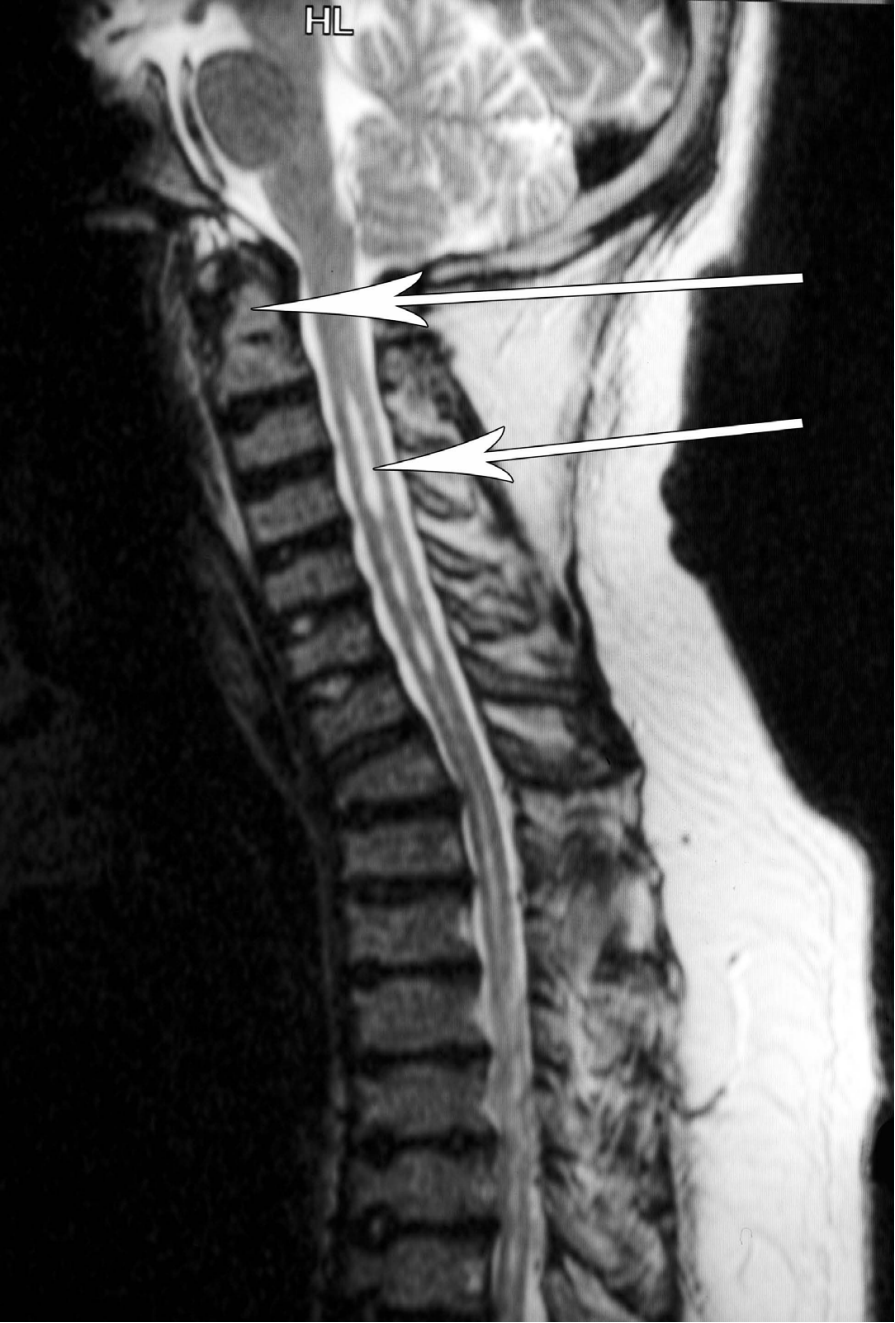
MRI cervical spine saggital section, with saturation band, anterior beaking of the cervical vertebrae and syrinx between C3 and C7 is noted. Arrows indicate odontoid peg and syrinx.
